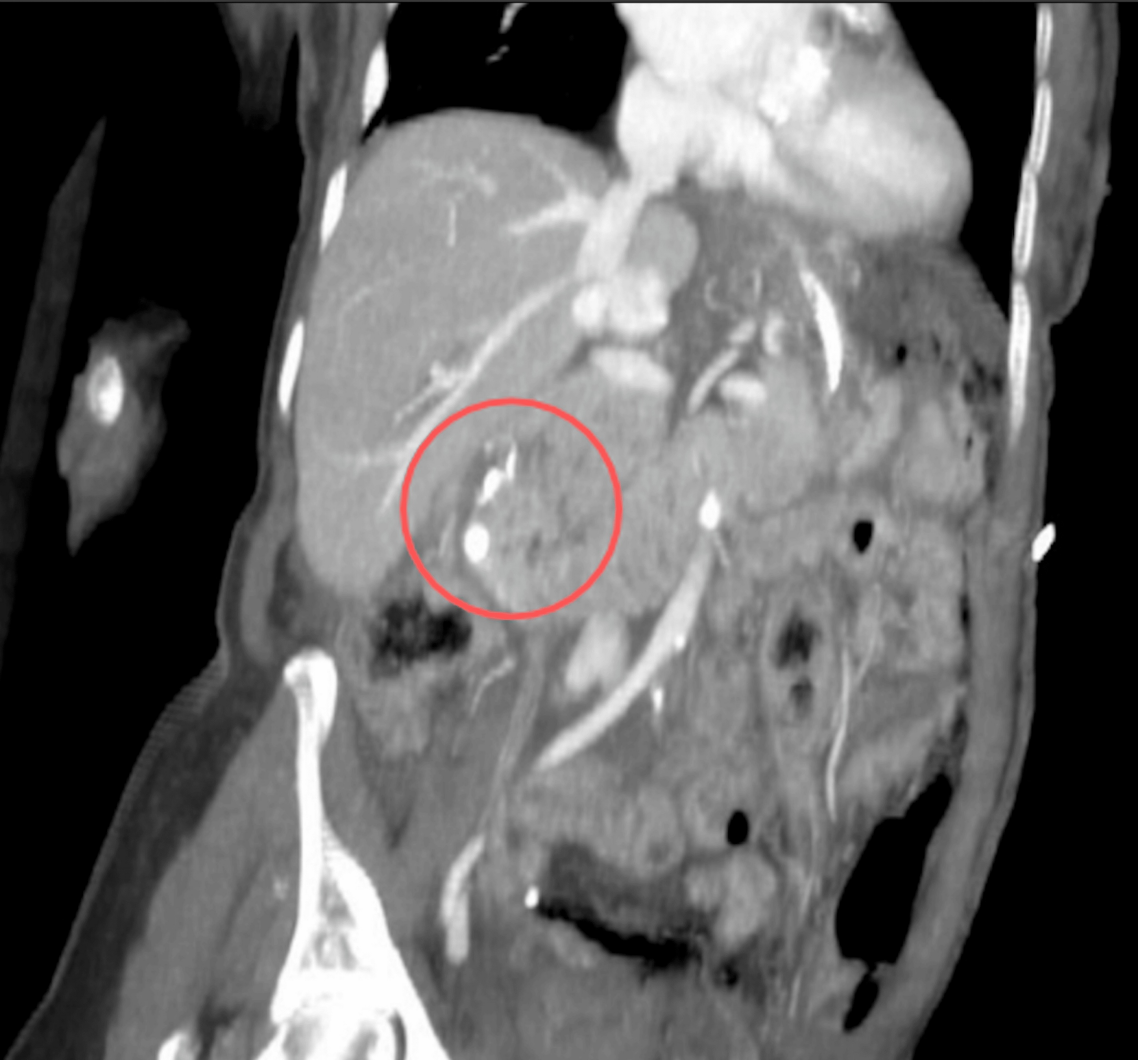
Oblique-angled computed tomography angiogram (CTA) of the abdomen and pelvis demonstrating the second and third portions of the duodenum, with the lateral staple line highlighted by the red circle.
